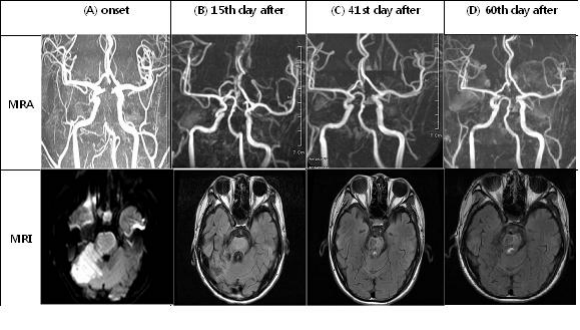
MRI scans and magnetic resonance angiographic findings before and after first injection of human umbilical cord blood (hUCB)-derived mesenchymal stem cells (MSC). (A) Partial recanalization of the basilar artery with residual severe stenosis was shown in the subacute infarction sites of bilateral pons, midbrain and right superior cerebellum. (B) Bilateral hypertropic olivary degeneration and further recanalization of the basilar artery with residual irregular stenosis was shown in the subacute to chronic infarction sites. (C) Luminal irregularity at the mid-to-distal basilar artery with residual stenosis was shown in the chronic infarction sites. (D) No intra-cranial lesion was shown in the chronic infarction sites, and effusion size was decreased at the right mastoid.
